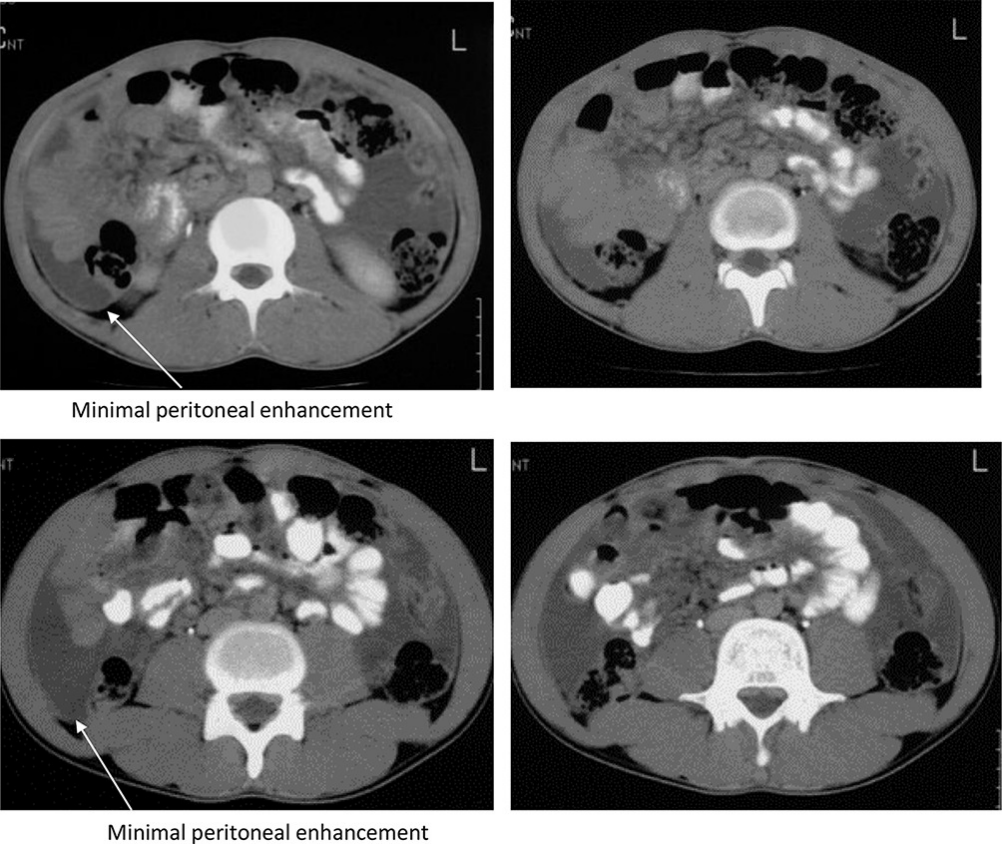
Axial CT images of a patient presenting with abdominal distension and pain showing ascites with minimal peritoneal thickening and enhancement. A diagnosis of tubercular peritonitis was made, following which an ascitic tap was performed. Culture of the ascitic fluid showed growth of *Mycobacterium tuberculosis*. Thus, the diagnosis of tubercular peritonitis was confirmed.
